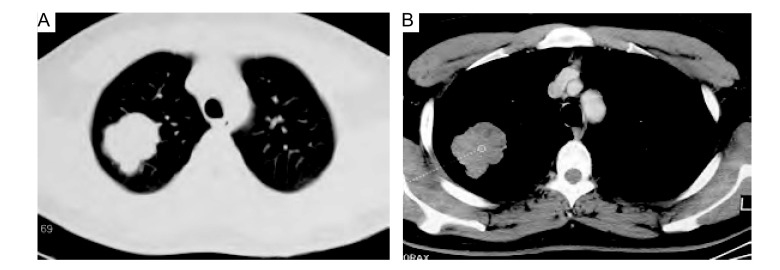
胸部CT。A肺窗和B纵膈窗示右上叶软组织团块影。 Chest CT. A pulmonary and B mediastinum windows CT showing a mass lies in right upper lung.
